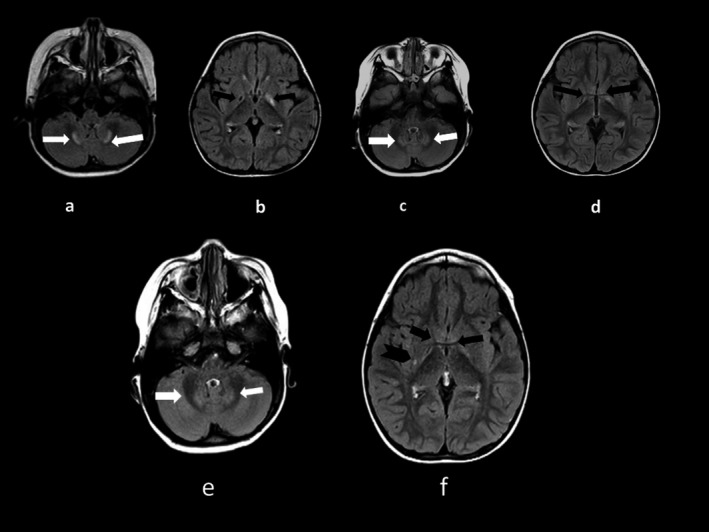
(a, b) T2‐FLAIR axial sections showing in the pallidus regions low signal intensity (cystic cavitation) in the right side and high signal intensity in the left side (black arrows); abnormal hyperintense signal in the dentate nuclei (white arrows) at 20 months. (c, d) corresponding sections, study confirming previous findings at 38 months. (e, f) T2‐FLAIR axial sections showing the same lesion of previous studies (white arrows), with an additional focal hyperintense lesion within the posterior striatum on right side at 3 years and 11 months (arrowhead)
